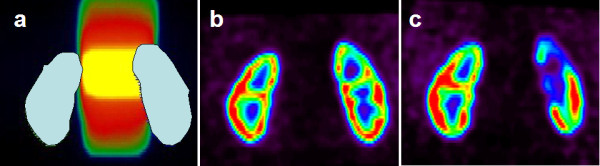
A preliminary qualitative example. Sample patient data from a pilot study at SCGH. The images are coronal slices, the grey shapes are the outlines of the kidneys. a) Planned radiotherapy dose distribution (yellow 45 Gy to black 0 Gy) and kidney volumes. b) Baseline (pre-treatment) SPECT intensity distribution (red - high activity, blue – low activity). c) 12-week post treatment SPECT showing reduced perfusion in the high-dose region.
